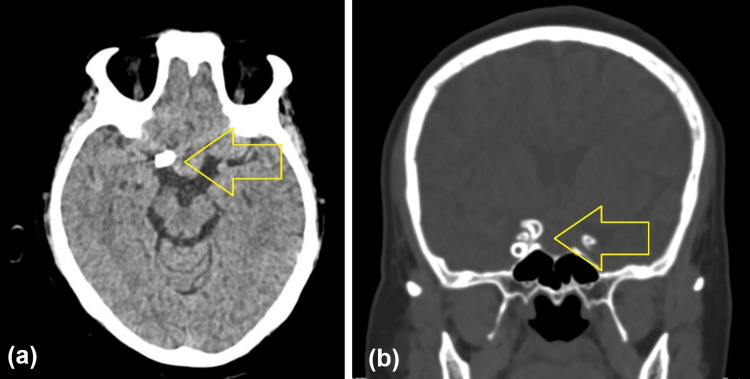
Post-treatment computed tomography (CT) of the brain. (A) Axial non-contrast CT demonstrating a hyperdense focus in the suprasellar region corresponding to the flow-diverter device, without hemorrhage (arrow). (B) Coronal non-contrast CT demonstrating the device in situ with no complications (arrow).
